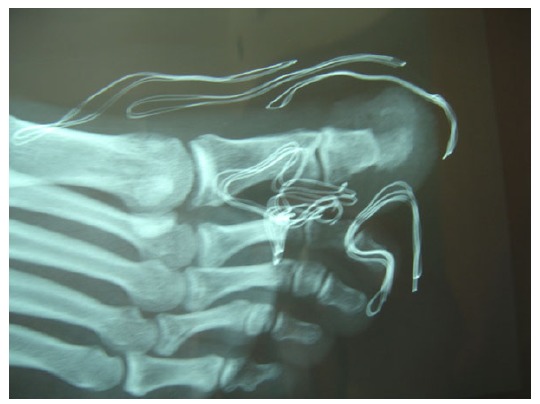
Postoperative Xray Lateral.
